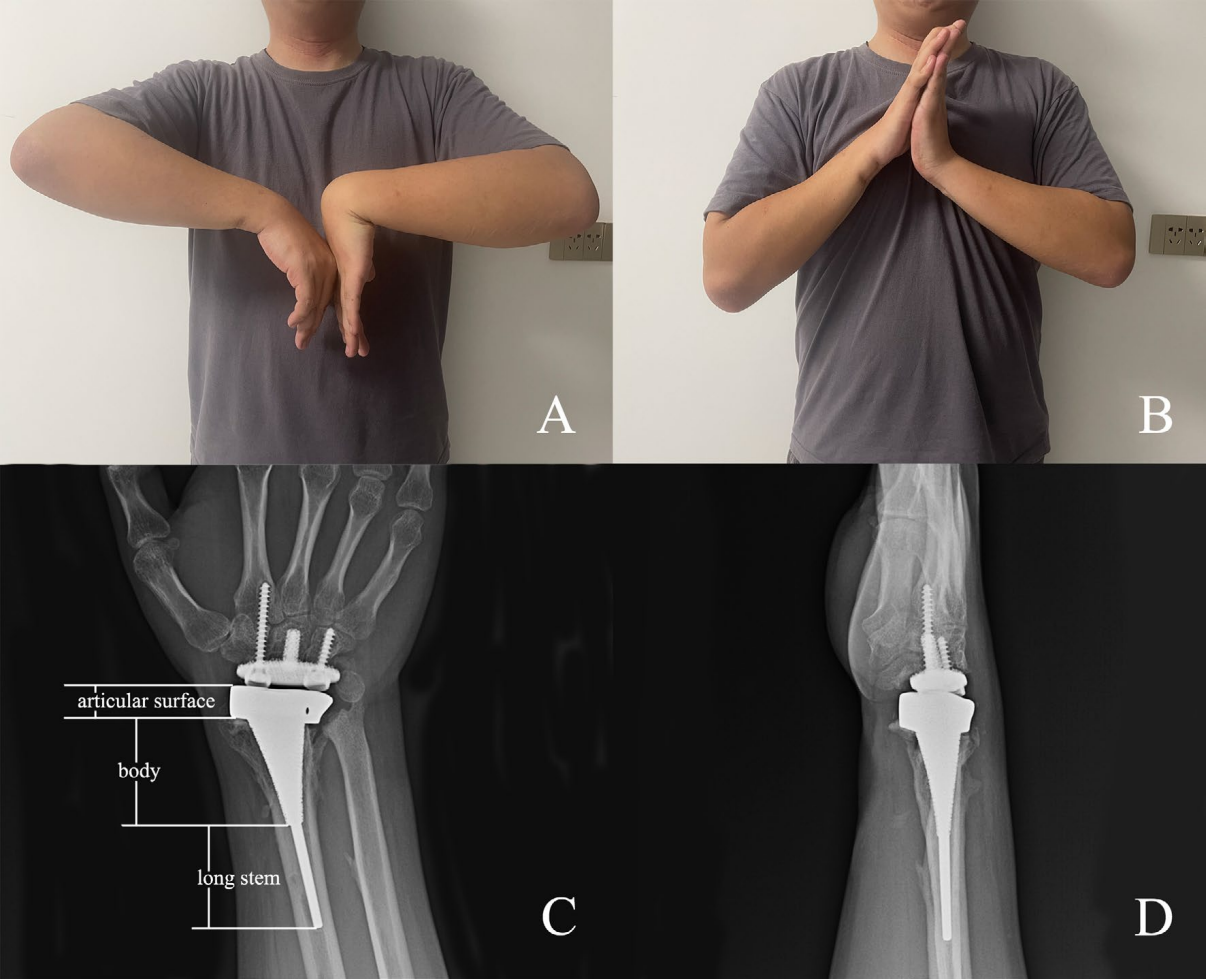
Postoperative range of motion and radiography of Patient 2 after 18 months. (A, B) Range of wrist flexion and extension. (C, D) The radiographic appearance of the prosthesis at the final follow‐up.
